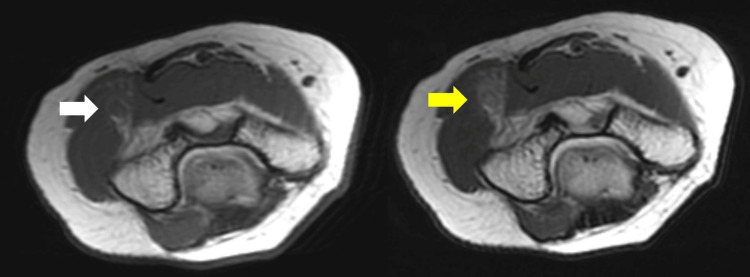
Axial view of preoperative MRI. Left side: T1WI. Iso- and high-intensity occupying lesions (white arrow) are seen. Right side: T2WI. High-intensity occupying lesions (yellow arrow) are seen. T2WI, T2-weighted image; T1WI, T1-weighted image
